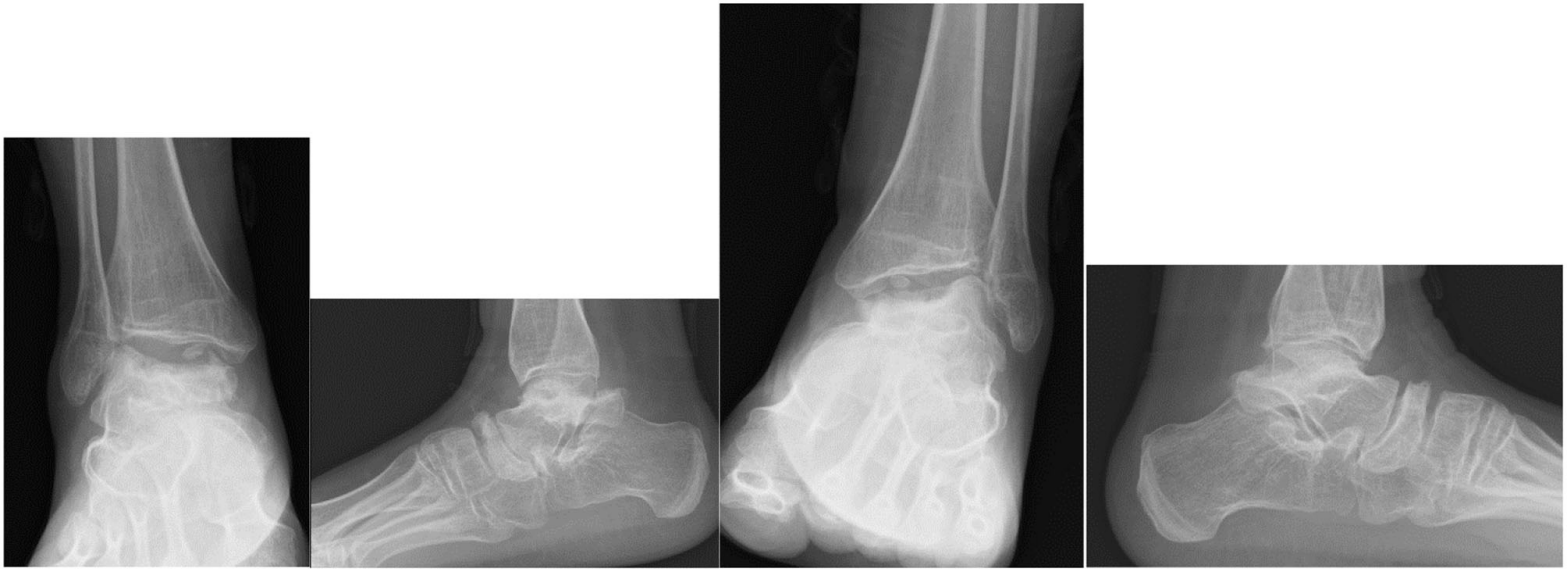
X-rays of both feet and ankles of a 20-year-old MPS IV patient with distal tibial epiphyseal dysplasia and valgus wedging of the tibial articular surface. Avascular necrosis, collapse and fragmentation of the talus and navicular can be seen bilaterally. Collapse of the 1st metatarsal head and arthritis of the 1st metatarso-phalangeal joint was also seen on the CT.
